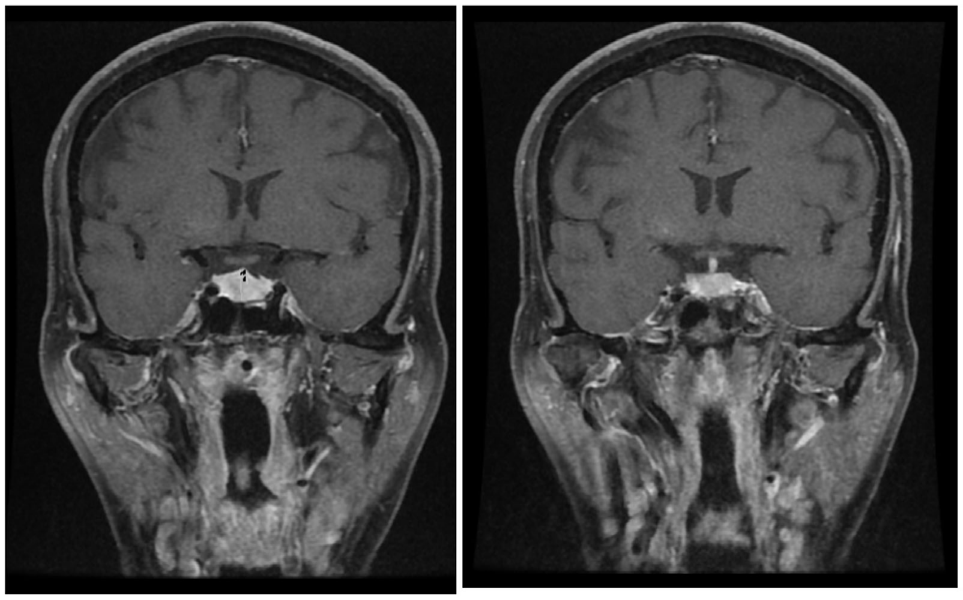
Follow-up MRI of a pituitary mass measuring 11 mm in height, which is near normal; the optic chiasm is seen to be free and the stalk is in the midline.
